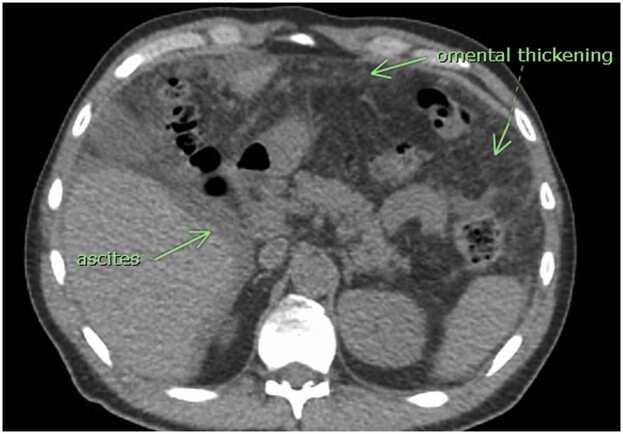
Axial CT of the abdomen demonstrating ascites and omental thickening.
